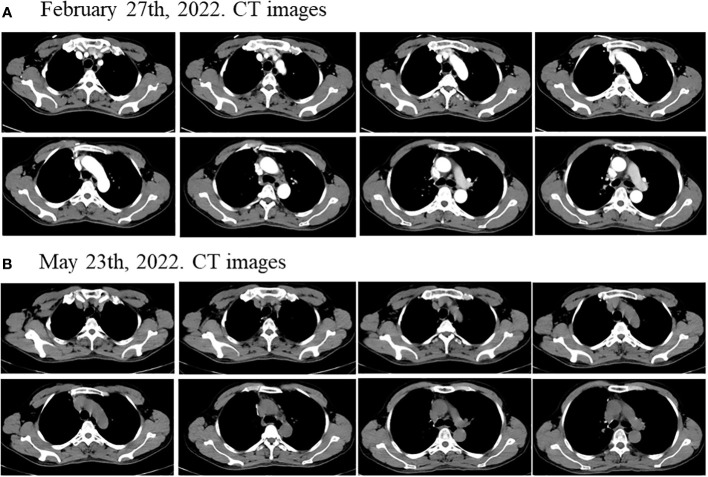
Disease status after surgical resection. The chest CT scan showed no evidence of malignant tumor recurrence about (A) 2.5 months and (B) 5.5 months after surgical resection.
